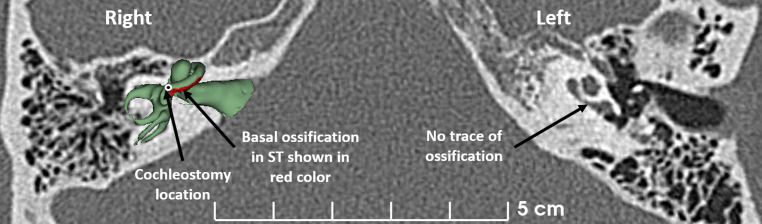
Three dimensional segmented inner ear image of the right ear overlaid on the CT image slice (3D slicer). It shows the basal ossification in red color and the cochleostomy location as pointed by the white circle around a black dot. The left cochlea shows no traces of ossification
